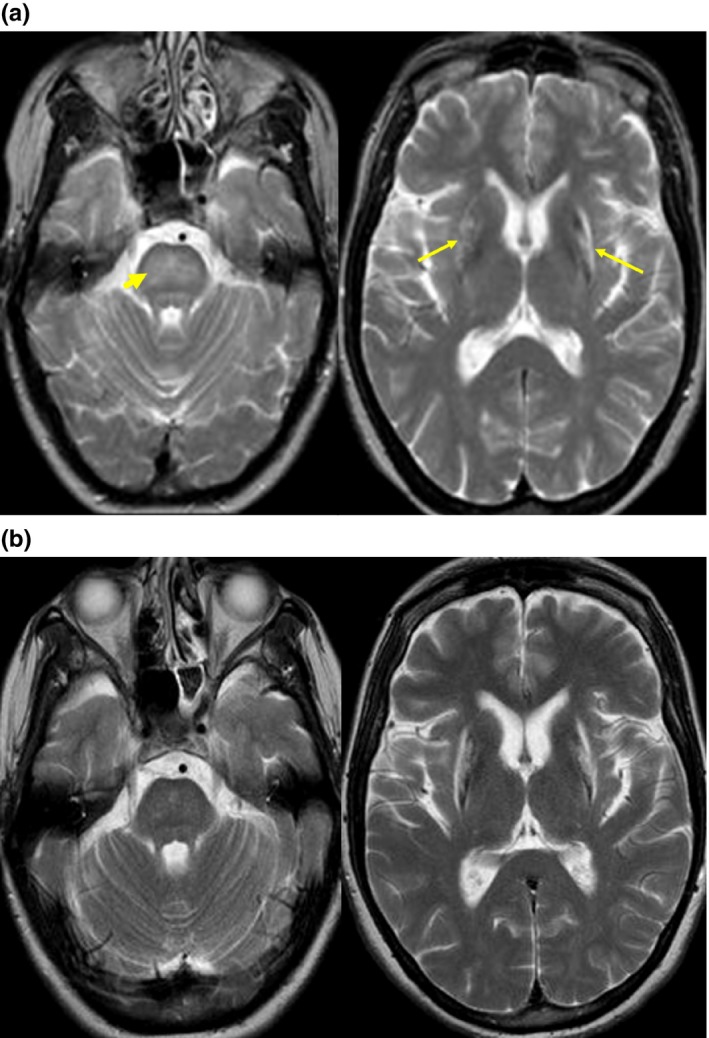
Neuroimaging improvement with brain edema reduction 3 months after starting treatment with ATTM and persistence of chronic necrotic lesions of basal ganglia. Patient 2. (a). Pre‐ATTM brain MRI. Increase in signal intensity on T2‐weighted images involving diffusely the pons (arrowhead), and the external border of lenticular nuclei (arrows). (b). Post‐ATTM brain MRI. Observe the almost complete resolution of the diffuse pontine signal abnormalities, but persistent T2 signal abnormalities associated with atrophy, likely related to necrosis, of both putamina
